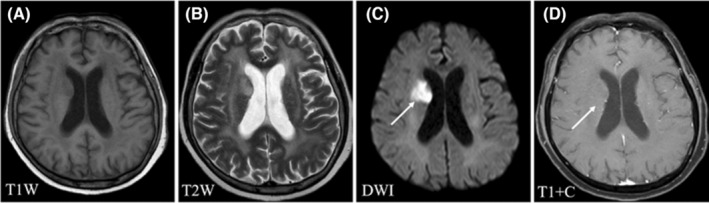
Pattern III of brain metastases in SCLC. Pattern III: A 71‐year‐old man without history of smoking was diagnosed with SCLC. A follow‐up head MRI examination revealed the presence of three intracranial lesions located in the right periventricular, callosum, and left centrum semiovale. One lesion (white arrow) was located in the right periventricular, with hypointensity in T1WI (A), hyperintensity in T2WI and DWI (B, C), and no enhancement (D). Cerebral infarction was initially diagnosed. Finally, BMs of SCLC were confirmed by cerebrospinal fluid examination and imaging findings during follow‐up. BMs, brain metastases; DWI, diffusion‐weighted imaging; MRI, magnetic resonance imaging; SCLC, small cell lung cancer.
